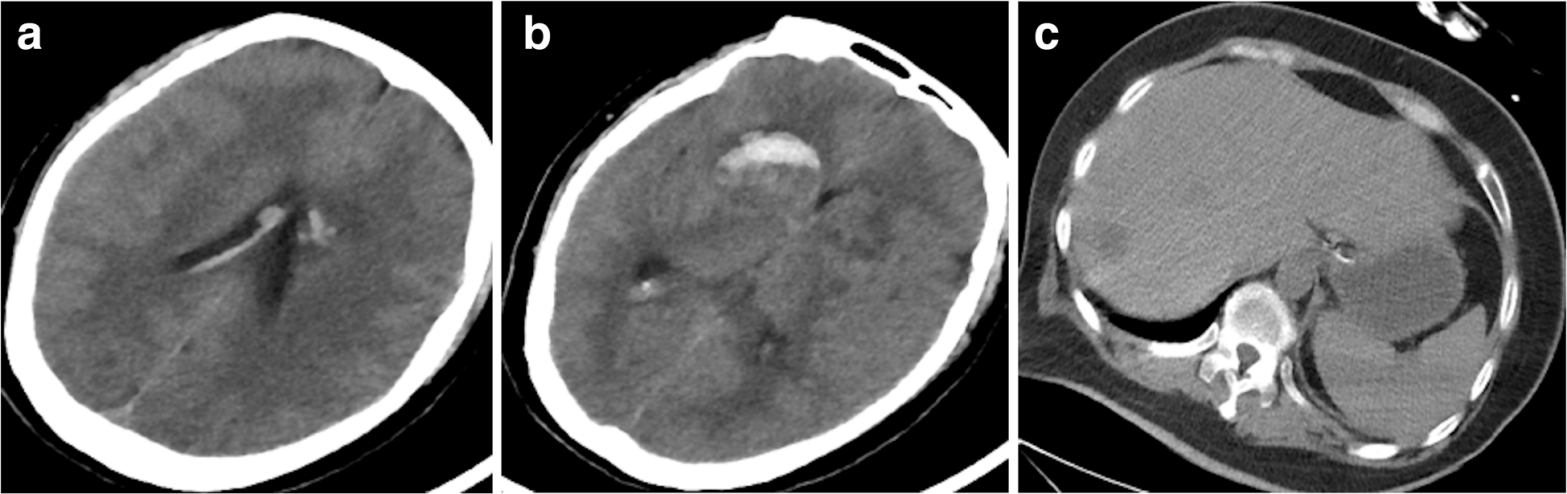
a and b: head CT scan showed bilateral basal ganglia hemorrhages and a subarachnoid hemorrhage; c: Abdominal CT scan demonstrating an area of abnormal attenuation measuring 18 mm × 14 mm in the right lobe of the liver
